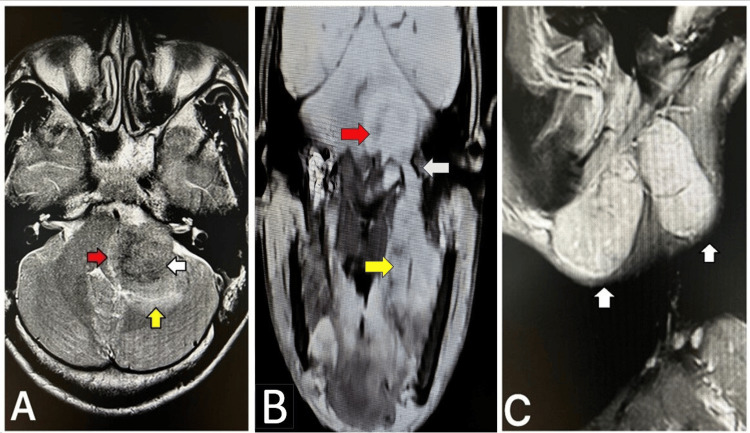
(A) T2-weighted axial image showing a left posterior fossa heterogeneous extraaxial lesion (white arrow) causing compression of the pons (red arrow) and cerebellum (yellow arrow). (B) T1FS contrast oblique-coronal image showing the enhancing left posterior fossa extraaxial lesion (red arrow) extending through the jugular foramen (white arrow) into the neck (yellow arrow). (C) T1FS contrast sagittal image showing enlarged enhancing left-sided neck lymph nodes (white arrows). T1FS: T1-weighted fat saturation
